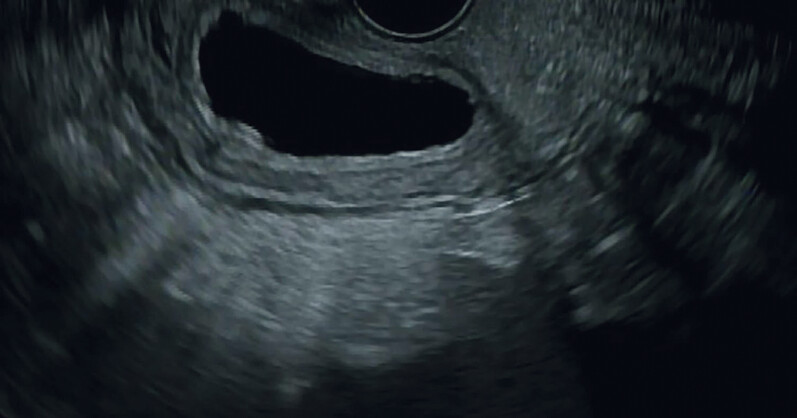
Endoscopic ultrasound demonstrating cyst with simple fluid content.
